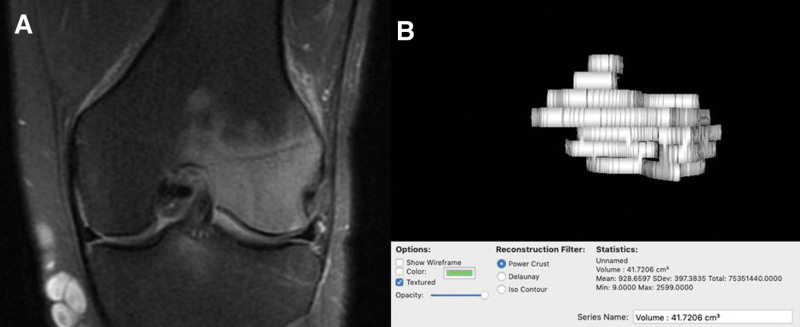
(A) MRI image of the knee with BME. (B) Measurement of BME 3D volume. BME = bone marrow edema, MRI = magnetic resonance imaging.
